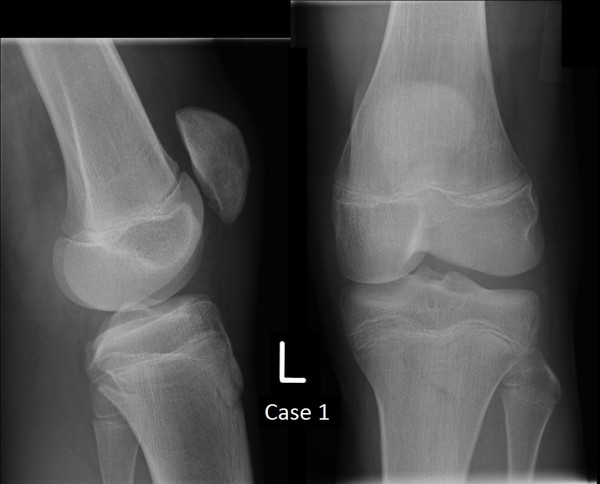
Plain film radiographs of Case 1 at 17 months.
